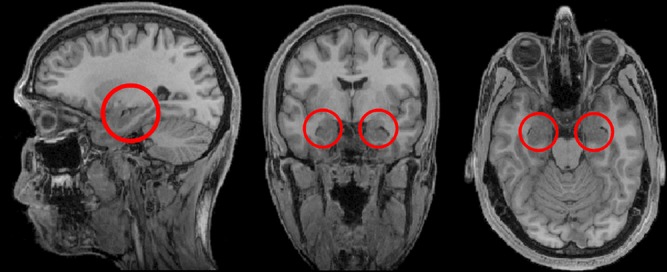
The hippocampi are circled in red on sagittal (left), coronal (middle) and axial views (right) from a T1-weighted structural magnetic resonance imaging (MRI) brain scan.
